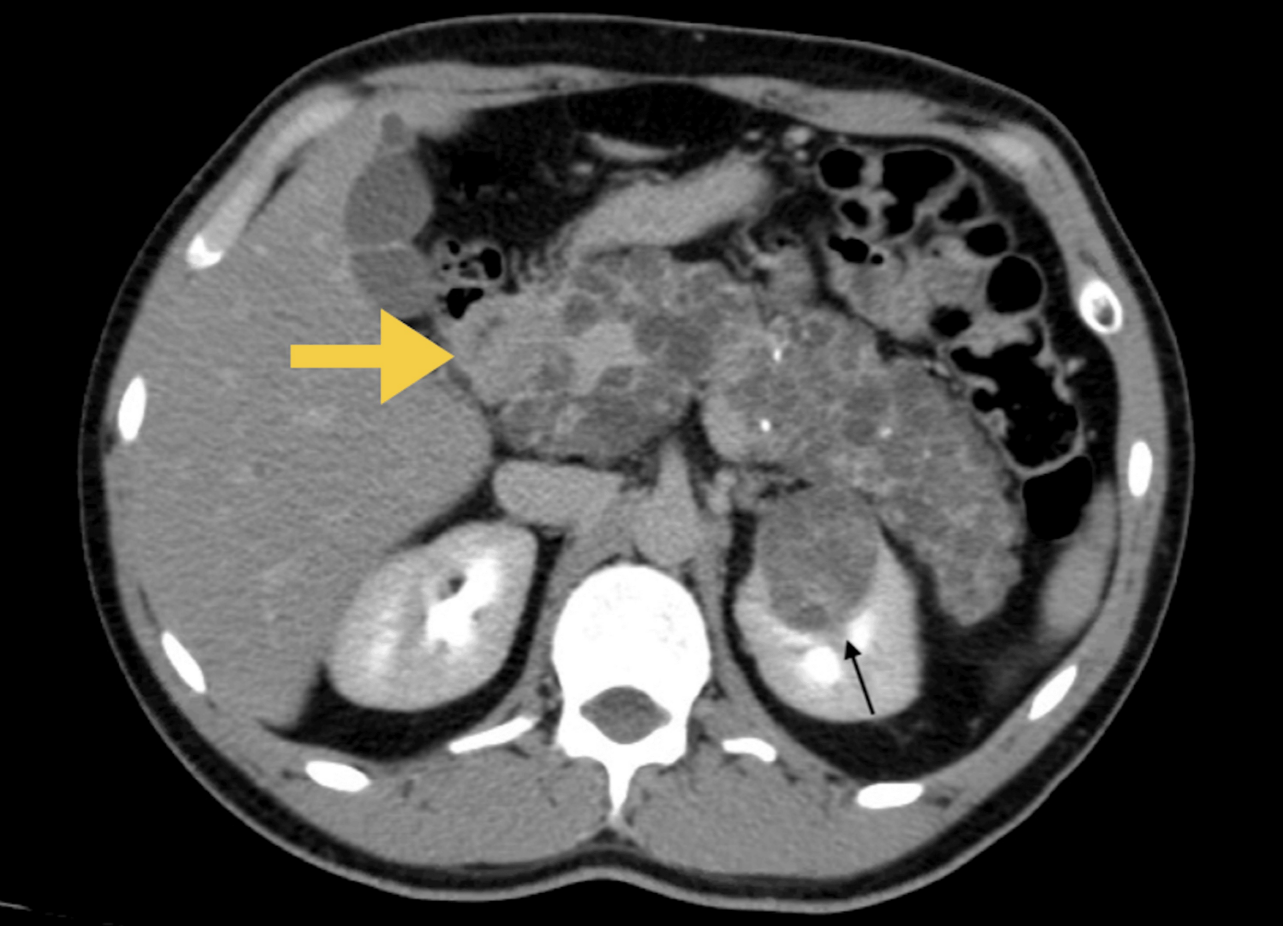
CT urography showing three complexes of multifocal RCC masses and pancreatic cyst/serous adenoma (axial view) CT: computed tomography, RCC: renal cell carcinoma
